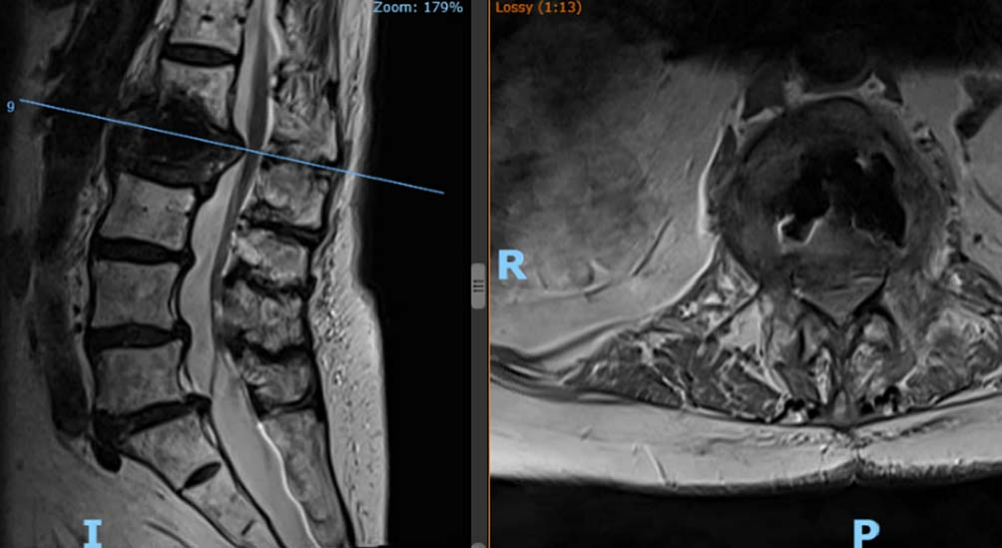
Patient’s lateral (left) and axial (right) MRI following the kyphoplasty procedure, demonstrating L1 fracture with retropulsion and compression of the conus.
